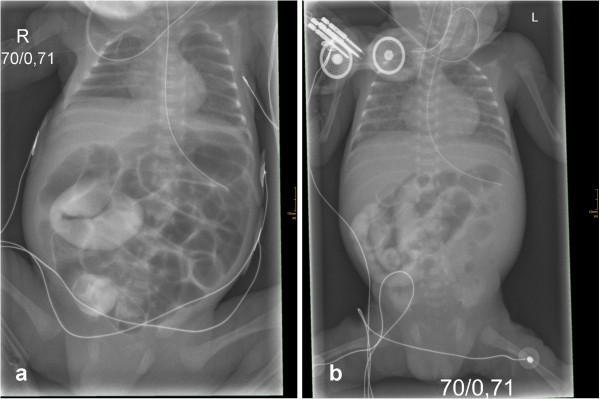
Initial X-rays of both twins. Chest and abdominal X-ray with contrast agent upon initial presentation of twin I (a) and II (b) showing contrast agent stopping at the inflated, distended small intestine next to signs of neonatal respiratory distress syndrome.
